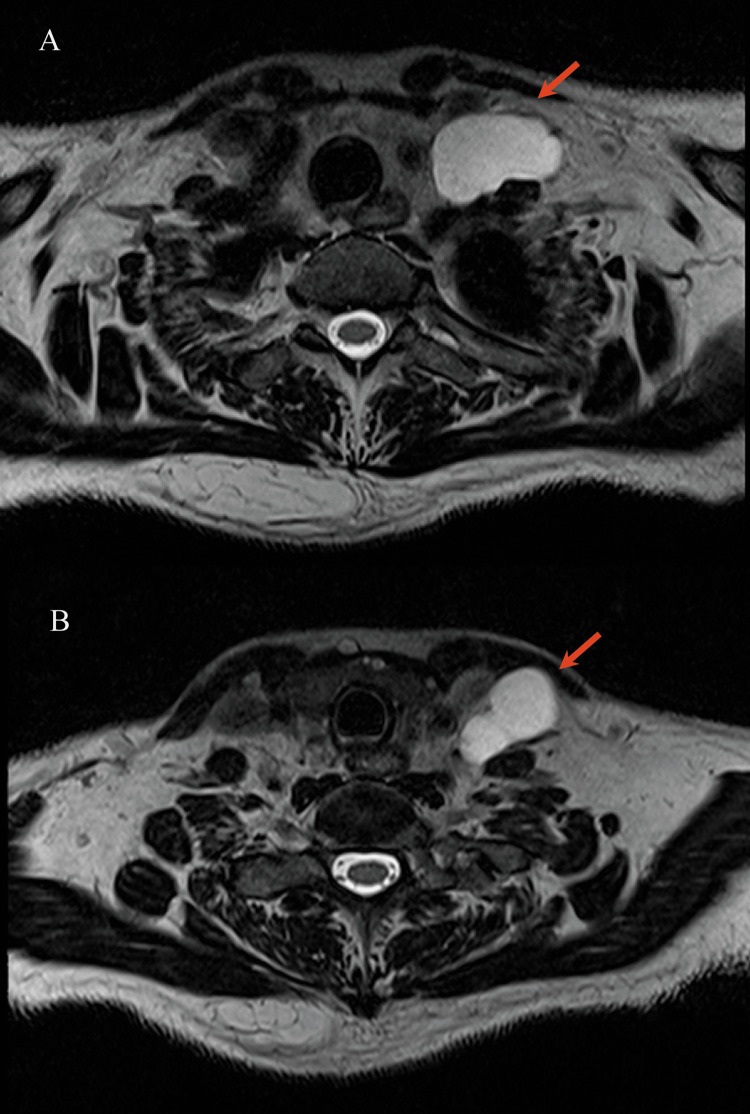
Contrast-enhanced MRI showing the cyst located on the right side behind the jugular vein and lateral to the thyroid gland (arrow). MRI: magnetic resonance imaging
